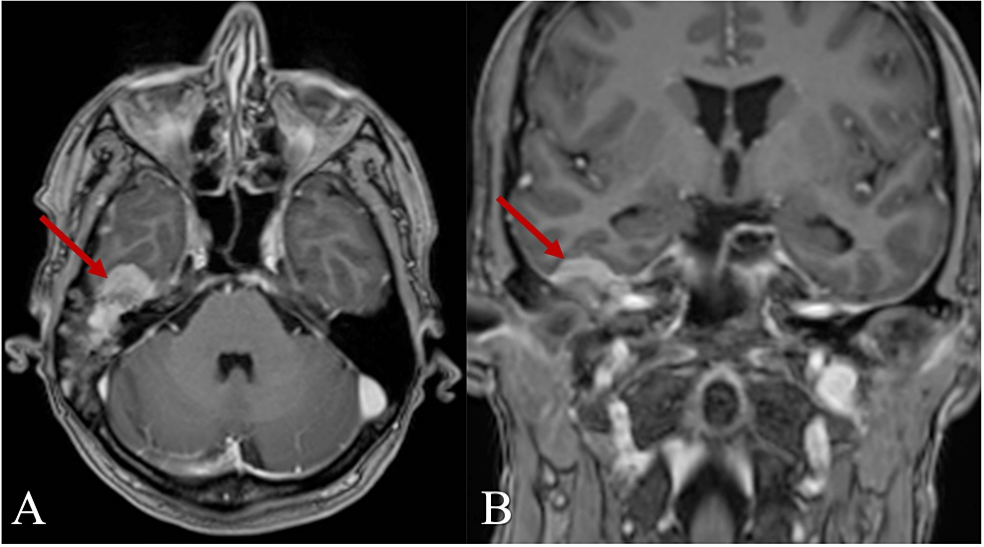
Head MRI of the relapsed tumor, T1-weighted axial (A) and coronal (B) views Arrows indicate a contrast-enhancing tumor extending intracranially into the temporal fossa and subtemporally.
